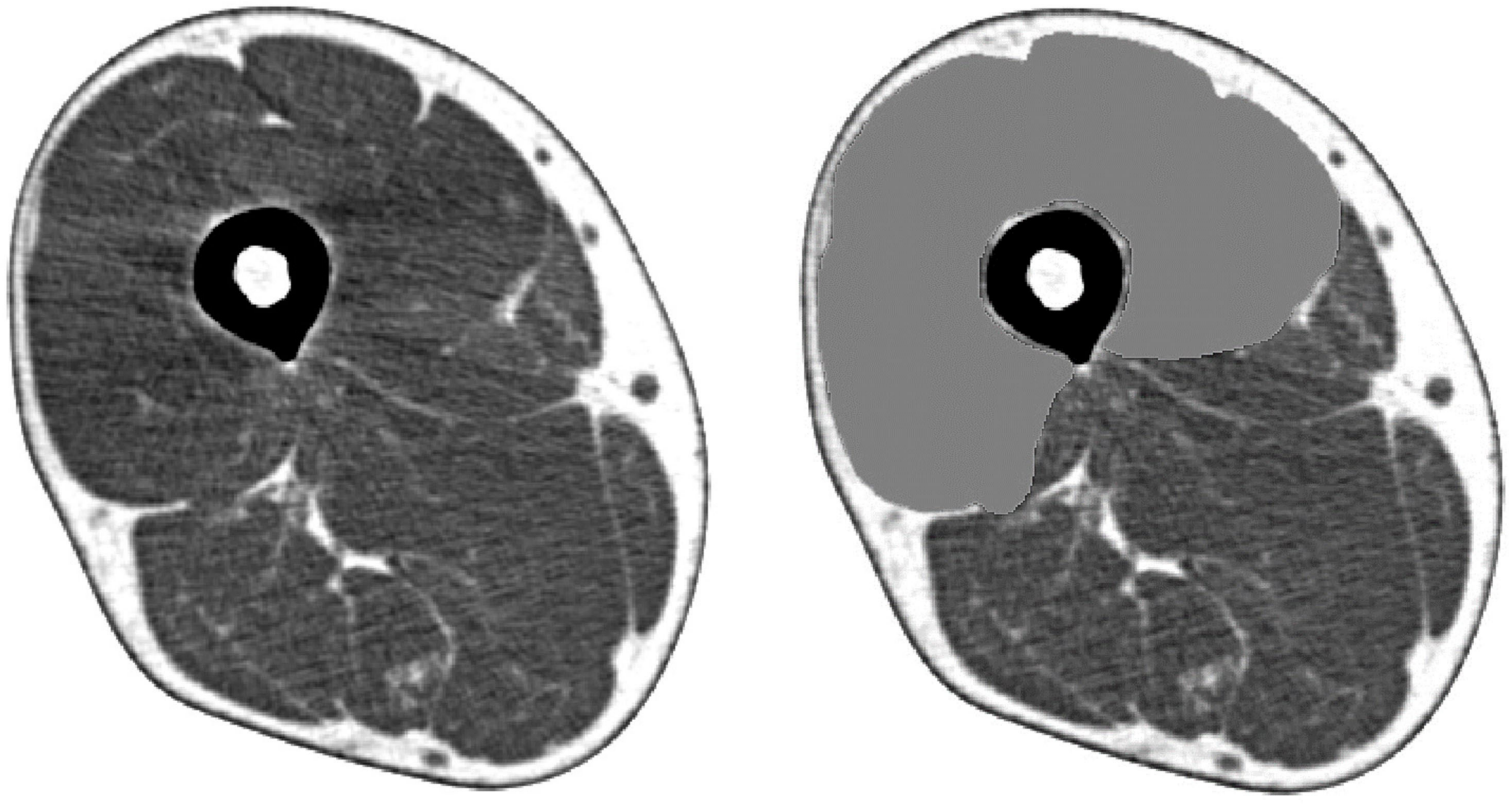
Cross-sectional muscle area of the quadriceps femoris determined by mid-thigh computed tomography.
